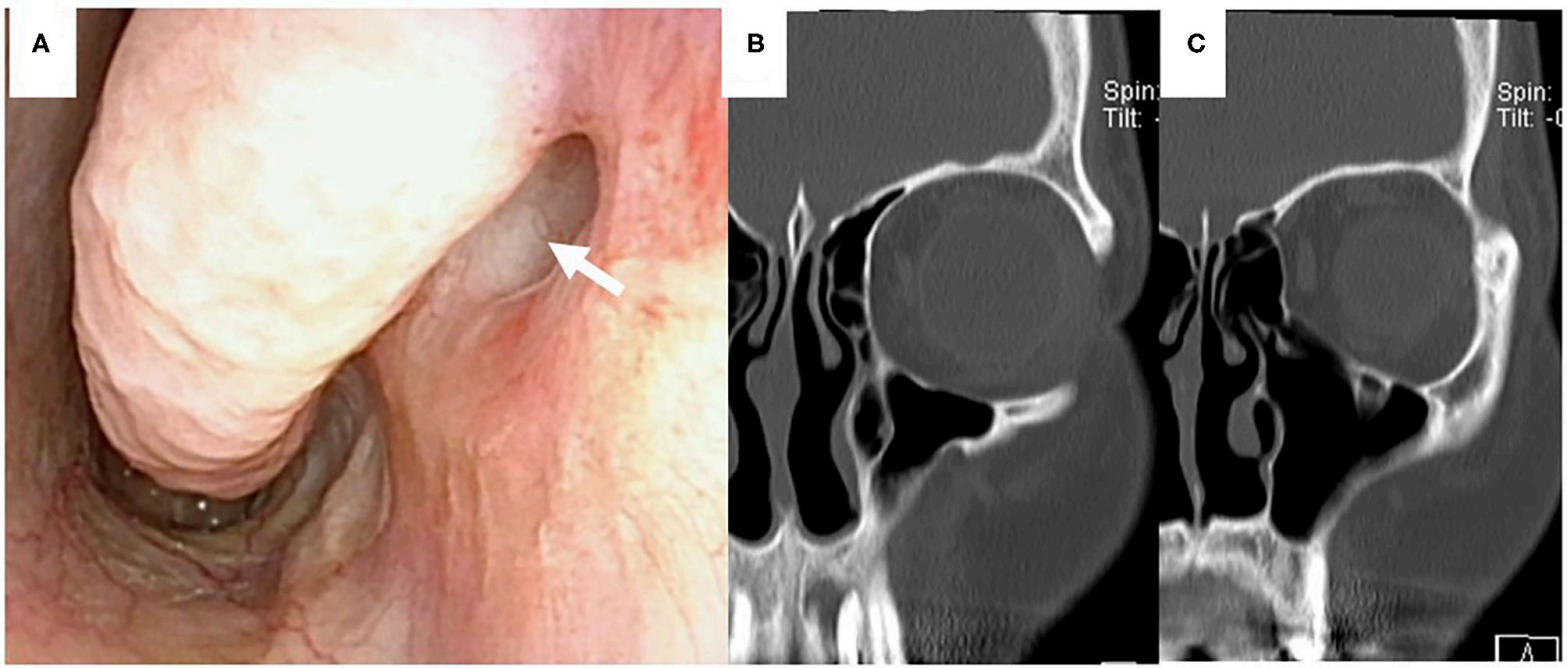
Post-operative findings 6 months after the operation. (A) Fiberscopic findings showed that the nasolacrimal orifice could be observed in the inferior meatus (white arrow). (B,C) Coronal CT scan findings showed the inferior meatus and patency of the nasolacrimal duct and orifice.
